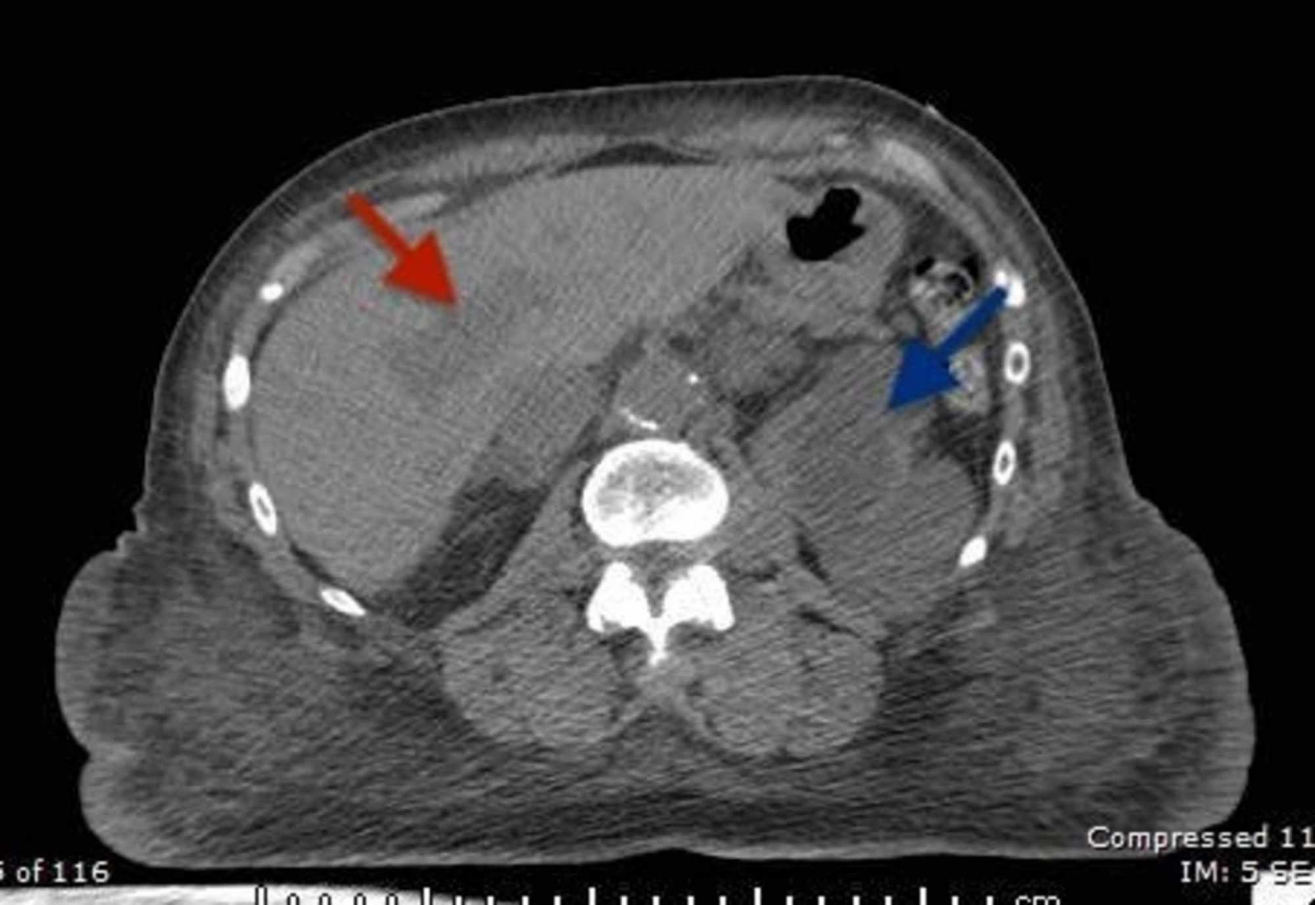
Axial CECT (contrast-enhanced computed tomography) scan of the abdomen showing metastasis to the liver (red arrow) and left adrenal gland (blue arrow).
